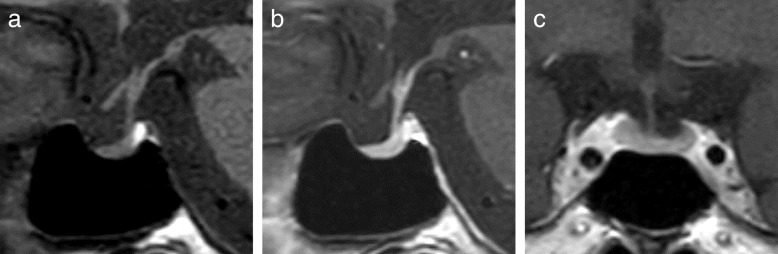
Magnetic resonance imaging of the pituitary gland (January 2018). a Sagittal T1-weighted plain magnetic resonance imaging (MRI) study showing normal high-intensity signals in the posterior lobe of the pituitary. b, c Gadolinium-enhanced MRI scans (b, sagittal plane; c, coronal plane) showing homogeneous enhancement of the normal hypophyseal stalk and mild atrophy of the anterior lobe of the pituitary gland. The width, length, and height of the pituitary gland are 16.2, 9.8, and 2.2 mm, respectively
